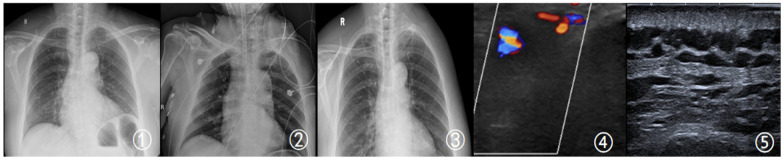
①: Preoperative 3-month DR image of the patient; ②: postoperative follow-up DR image; ③: postoperative 3-month follow-up DR image; ④: preoperative color Doppler ultrasound image, with no vascular abnormalities detected; ⑤: postoperative 1-year follow-up color Doppler ultrasound image of the patient: patent blood vessels, with no abnormalities detected.
